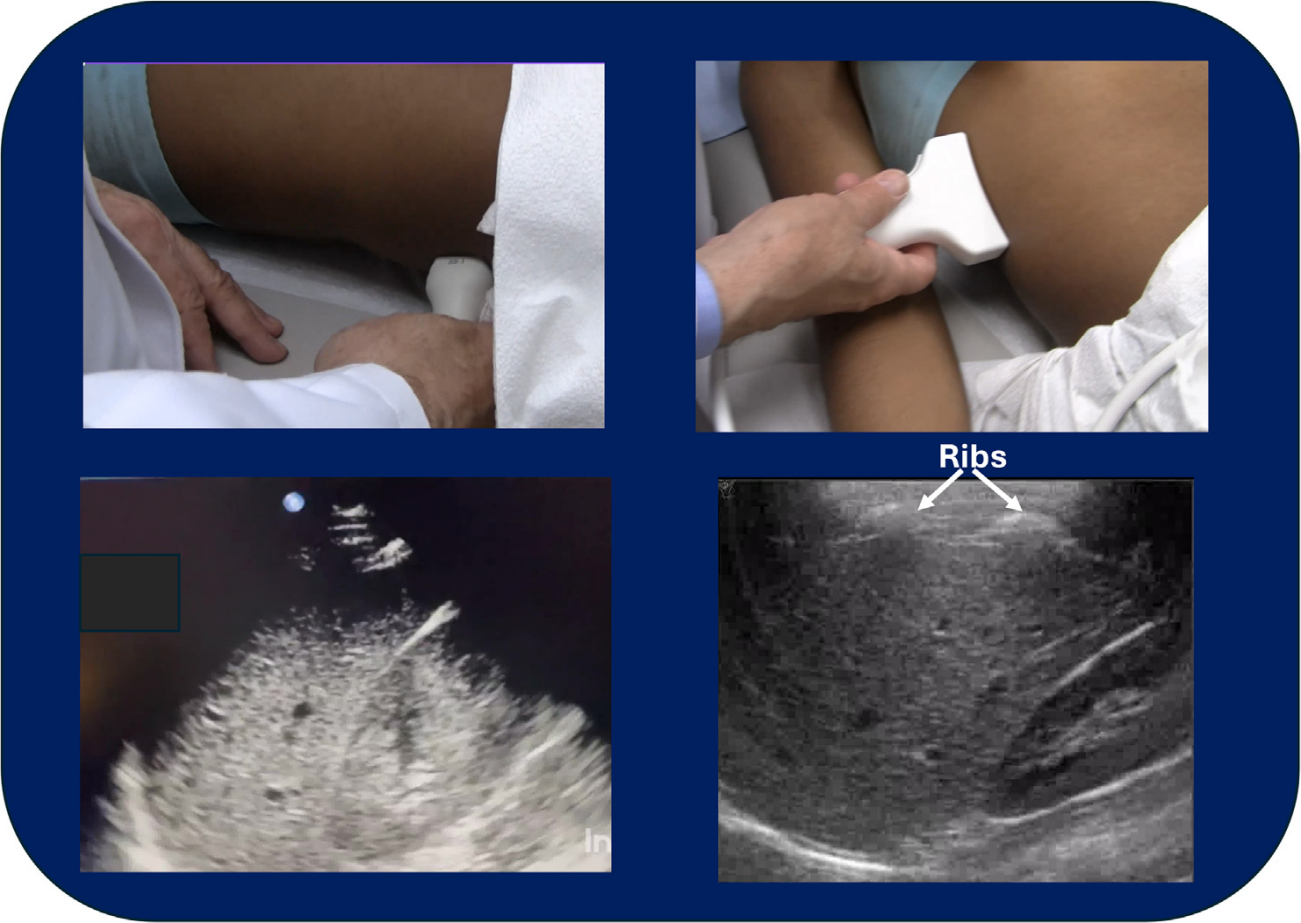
Comparison of images of standard eFAST views of the right upper quadrant. The probe placement photos in the upper half of the figure demonstrate the differences between the probe positions needed to obtain clear views of the right upper quadrant using as standard curved linear ultrasound probe (Phillips IU22 ultrasound system) and the K3900 computer echo tomography system and the concave 3 Mhz MAUI transducer. The lower half of the figure are the images obtained using the 2 systems. Again, with the K3900, the ribs can be easily identified but without the shadowing that would be expected with conventional ultrasound.
